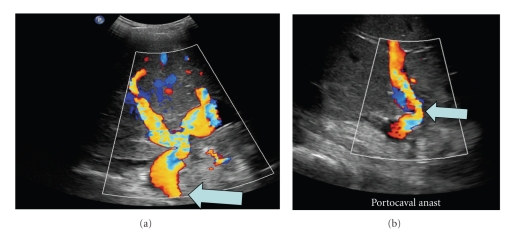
Ultrasonography documenting excellent flow through the cavoportal hemitransposition anastomosis (see arrow).
